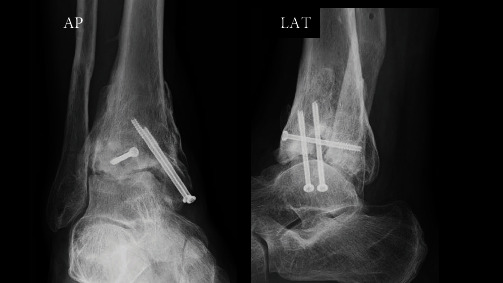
X-ray 4 years after surgery. Degenerative changes were observed in the articular surface (Takakura ankle osteoarthritis classification: Stage 2).
